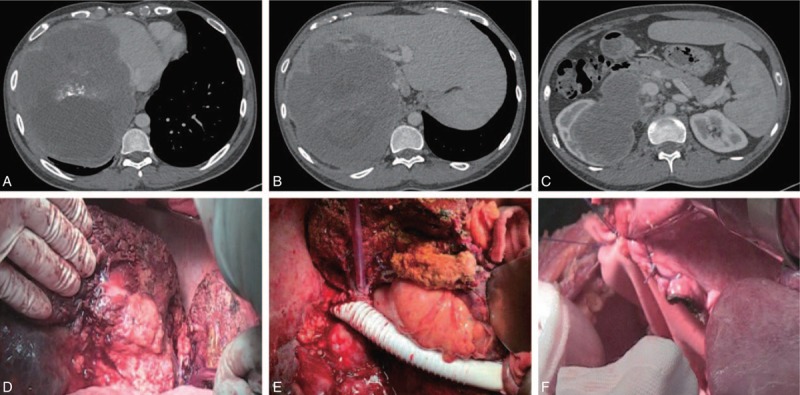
(A–C) Enhanced CT indicated a huge mass of 18 cm in diameter, which was in the right lobe of the liver with IVC and the right kidney invasion. The black arrays: IVC. The white array: portal vein. (D) The parasitic lesion in the right liver. (E) Repair the diaphragm with Dacron patches. (F) Reconstruct the IVC by an end-to-end anastomosis with an ePTFE graft, the array: ePTFE graft.
